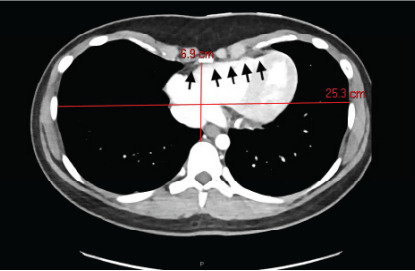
Contrast-enhanced chest computed tomography (axial plane) demonstrating right ventricular compression from severe pectus excavatum (black arrows). Thoracic cavity measurements are included in red to highlight the abnormal anterior-posterior diameter of this patient’s chest wall.
